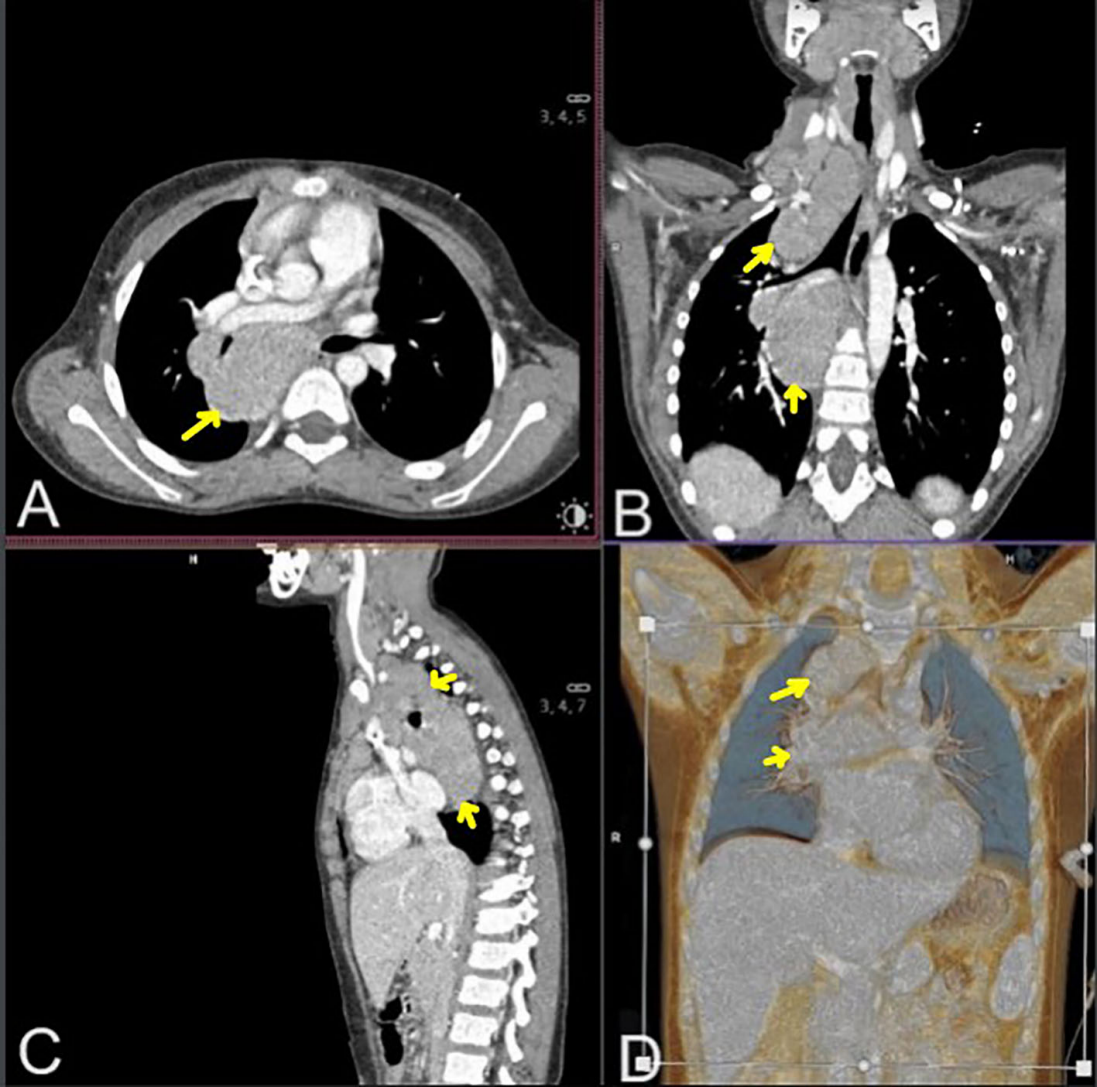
Chest and neck computed tomography scans in mediastinal window after contrast agent administration: transverse (A), coronal [(B) multiplanar reconstruction, (D) volume rendering technique reconstruction], and sagittal (C) section. Neck and mediastinal Hodgkin lymphoma masses are marked with yellow arrows.
